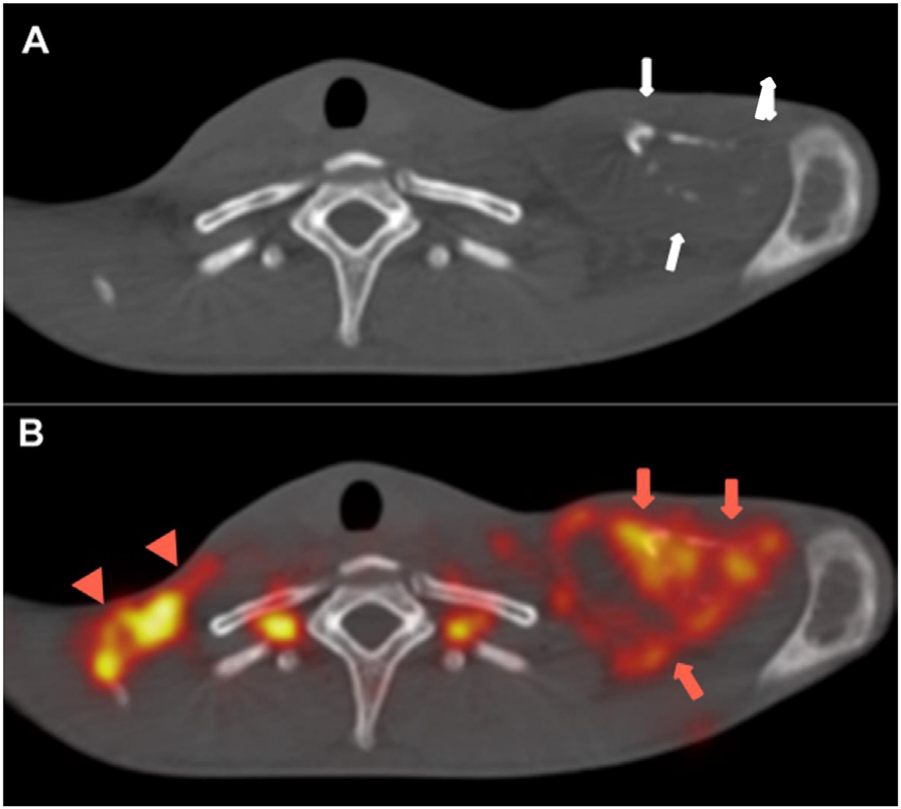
(A) Axial CT image (bone window) showing large erosions of the left clavicle with soft tissue swelling (white arrows). (B) Axial fused FDG PET/CT image showing FDG uptake (red arrows) of the lytic lesions in the left clavicle and in the fluid collection around the bone (SUVmax = 14.3). There is also intense uptake in the right supraclavicular lymph nodes (arrowheads).
